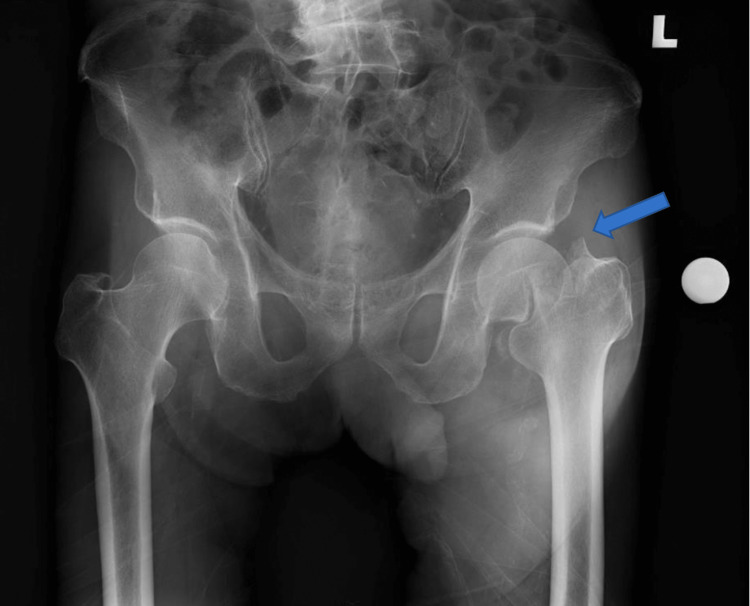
AP radiograph showing a left neck of femur fracture (arrow).
